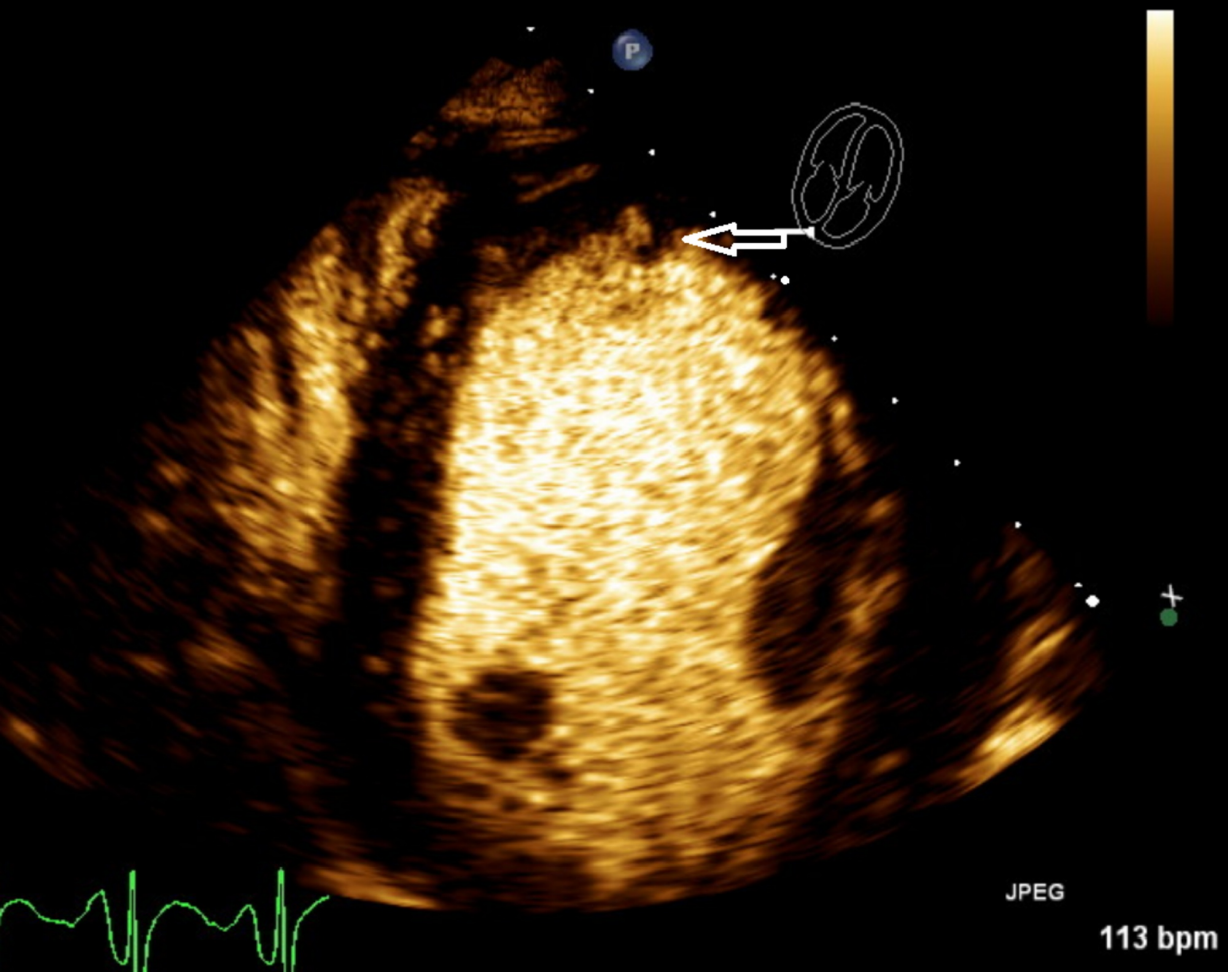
Patient 2 Echocardiogram
